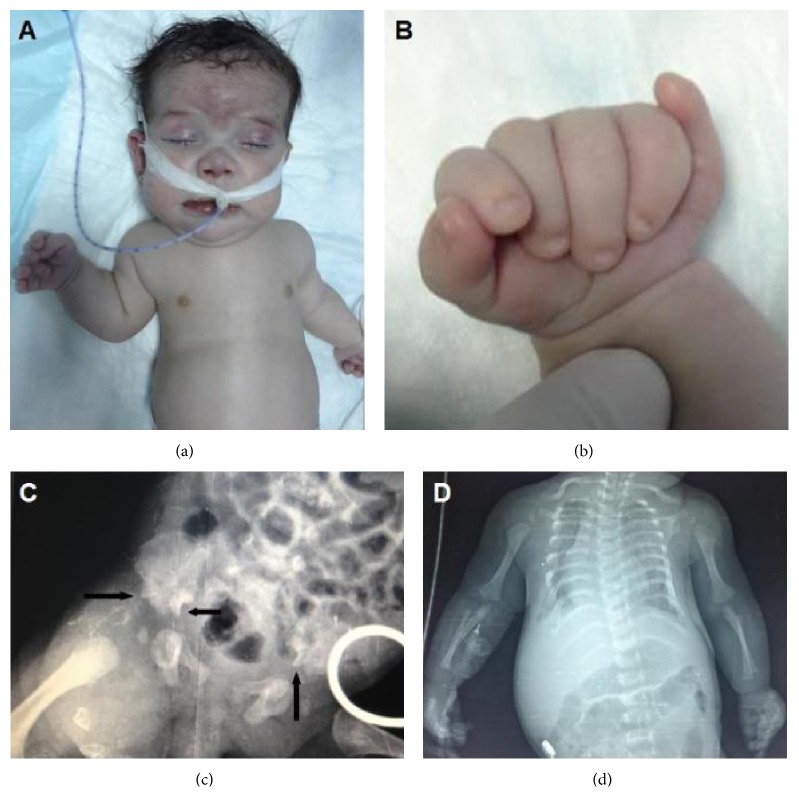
Clinical and X-ray features of the baby: (a) characteristic facial features of prominent forehead, low-set ears, narrow thorax, short upper extremities, a short and flat nose, and micrognathia; (b) polydactyly and hypoplastic nails of the hands; (c) trident aspect of the acetabular roof, ossification defects at the inferior aspect of the lateral iliac margin; (d) narrow thorax, short ribs, and high clavicle bones.
